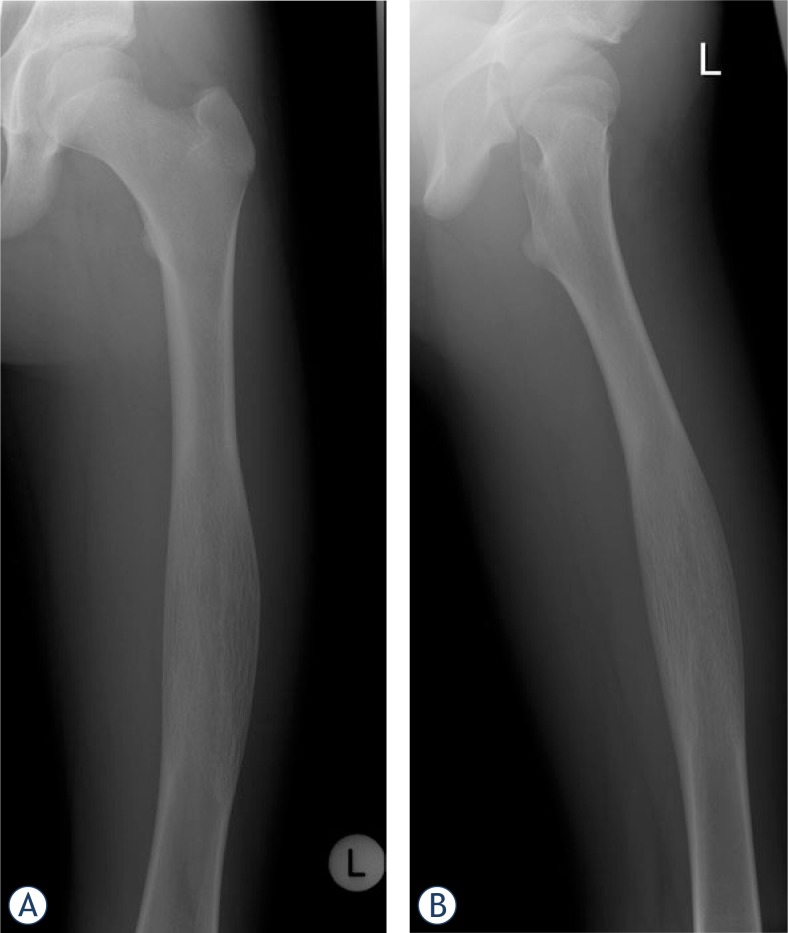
X-ray shows a 12 cm intraosseous expansion and lamellated periosteal reaction in the diaphysis of the left femur (anterio-posterior, lateral).
